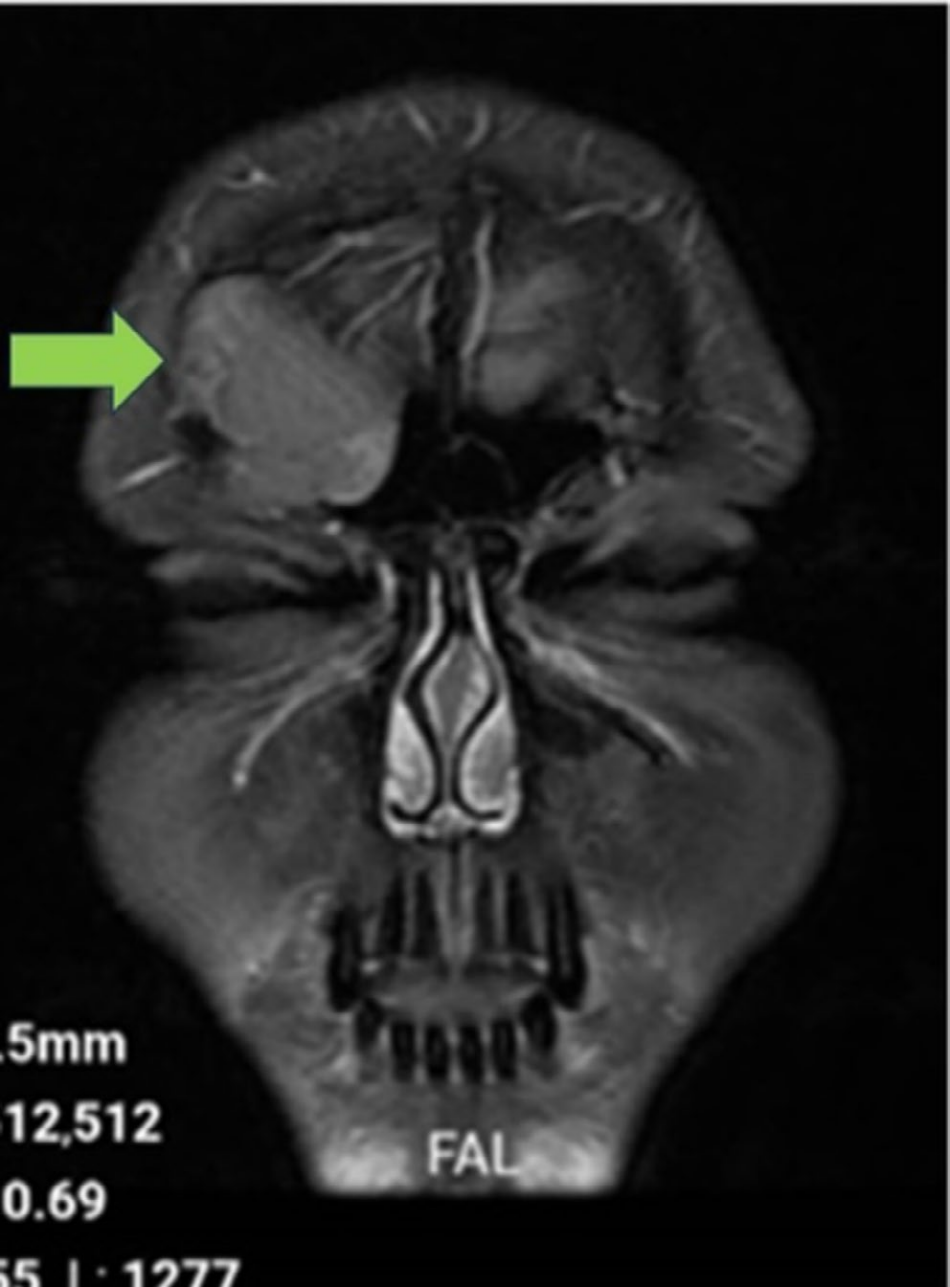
Coronal gadolinium‐enhanced T1‐weighted MRI showing absence of enhancement, indicating a non‐vascular lesion consistent with an epidermoid cyst.
